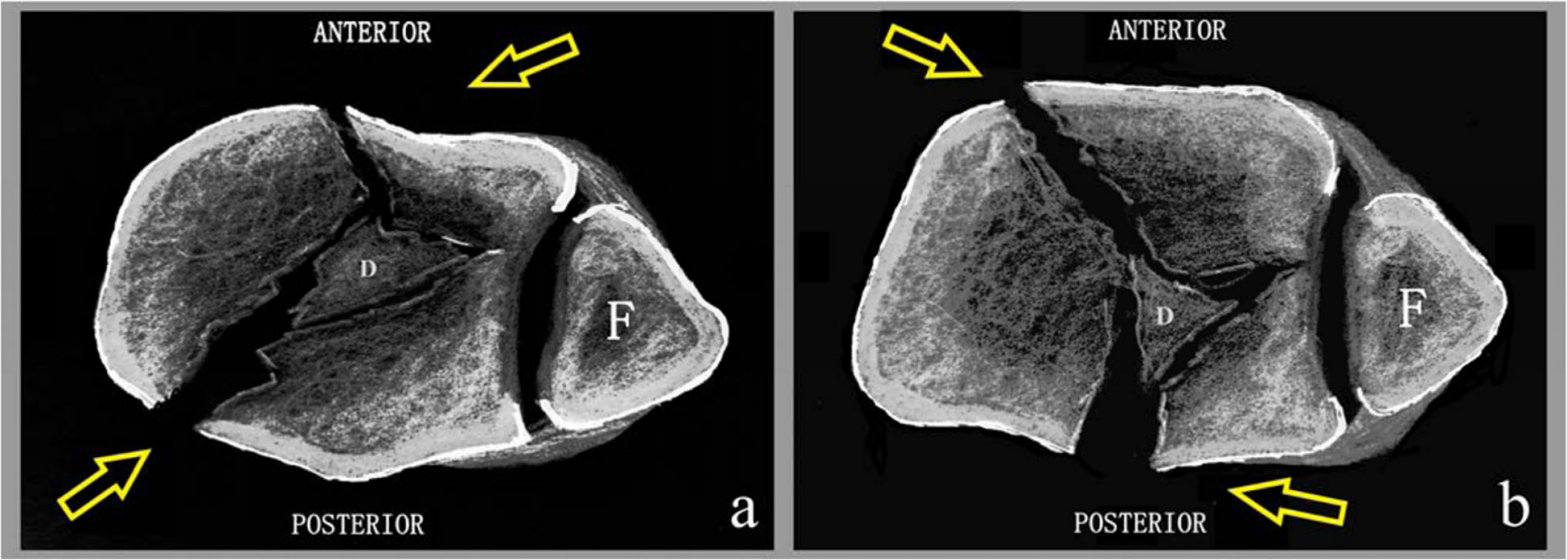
The surgical approach is established according to the fracture line and the displacement of the fracture fragments shown by CT scan 1 cm above the ankle joint surface. a The posterior malleolar fragment involves the tarsal tunnel, and the die-punch fragment is located laterally, using the posteromedial approach combined with the anterolateral approach. b The posterior malleolar fragment does not involve the tarsal tunnel, and the die-punch fragment is located medially, using the posterolateral approach combined with the anteromedial approach. The anterior approach is adjusted according to the position of the die-punch fragment and fracture line.
